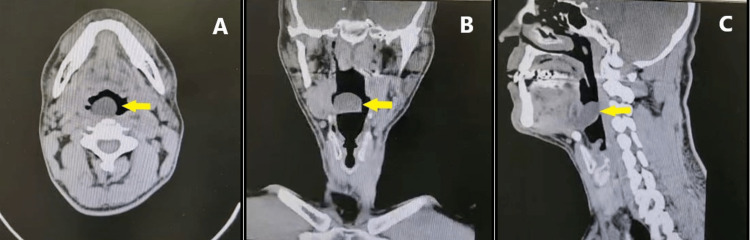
A preoperative computed tomography scan in the axial (a), coronal (b), and sagittal (c) planes of the head and neck showing a well-defined, 3 × 2 cm, hypodense cystic lesion on the vallecula (yellow arrow)
